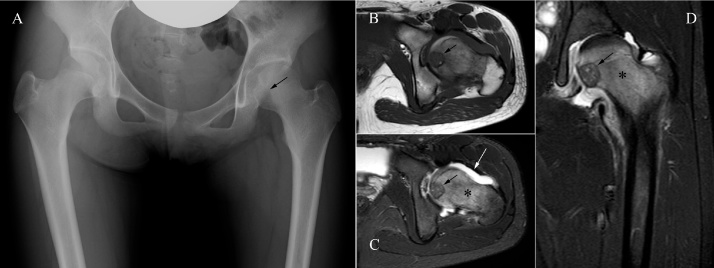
A radiograph (A) and MR images (B–D) at first presentation; B: T1-weighted axial image; C: STIR axial image; D: STIR coronal image. The tumor was located just underneath the joint cartilage (black arrows), and was surrounded by extensive oedema (*) and accompanied by hip joint effusion (white arrow).
